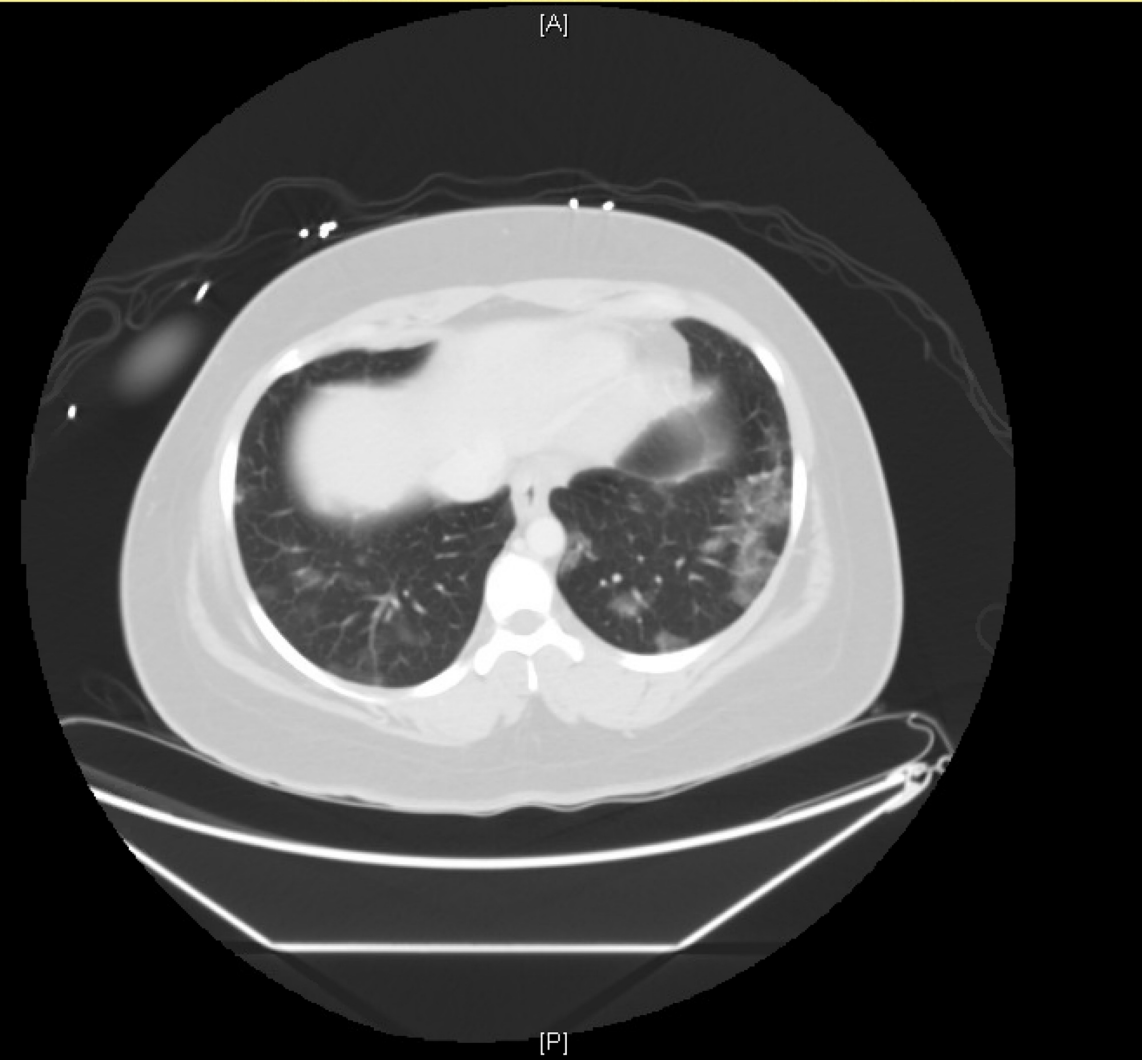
CT Abdomen/Pelvis basilar lungs demonstrating peripheral ground glass opacities (GGOs).
